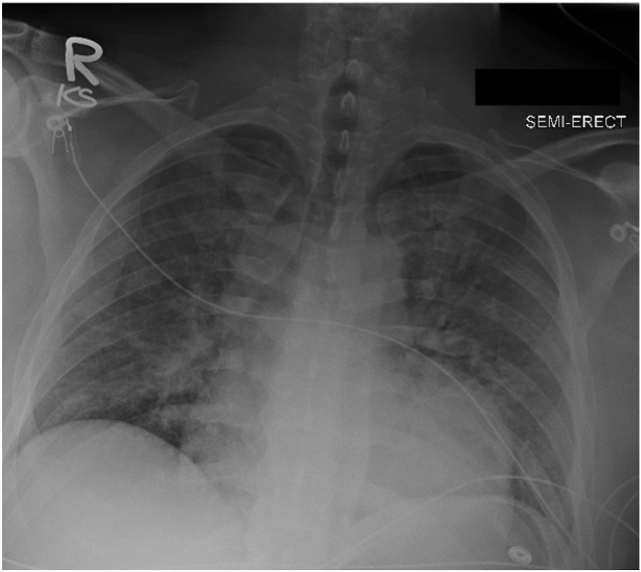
Single-view anteroposterior chest radiograph demonstrates diffuse bilaterial interstitial and alveolar opacities consistent with pulmonary edema.
